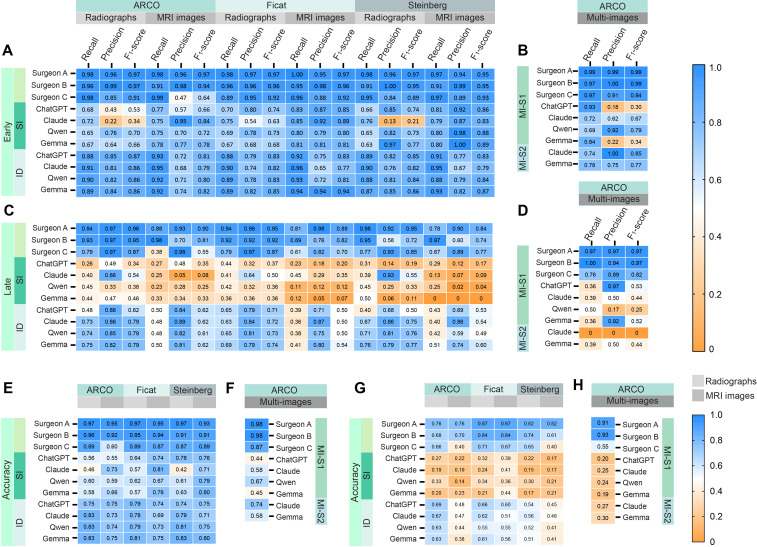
Early- and late-stage osteonecrosis of the femoral head (ONFH) differentiation and detailed grading accuracy. (A) Performance metrics (precision, recall, *F*_1_-score) for early ONFH differentiation (single image [SI], image plus radiology description [ID]). (B) Performance metrics for early ONFH differentiation (multi-image setup 1 [MIS1], multi-image setup 2 [MIS2]). (C) Performance metrics for late ONFH differentiation (SI, ID). (D) Performance metrics for late ONFH differentiation (MIS1, MIS2). (E) Accuracy of early- and late-stage ONFH differentiation (SI, ID). (F) Accuracy for early and late differentiation (MIS1, MIS2). (G) The detailed accuracy of surgeons and multimodal large language models (MLLMs) in grading ONFH differentiation in the SI and ID configurations. (H) The detailed accuracy of surgeons and MLLMs in grading ONFH differentiation in the MIS1 and MIS2 configurations. ARCO: Association Research Circulation Osseous; MI: multi-image; MRI: magnetic resonance imaging.
